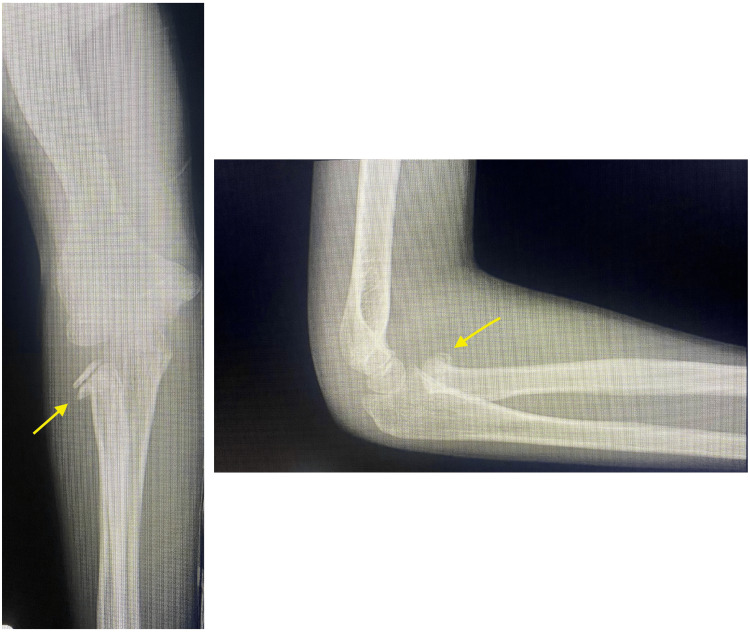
A simple radiograph revealed a fracture of the right radial neck (Judet type II).
